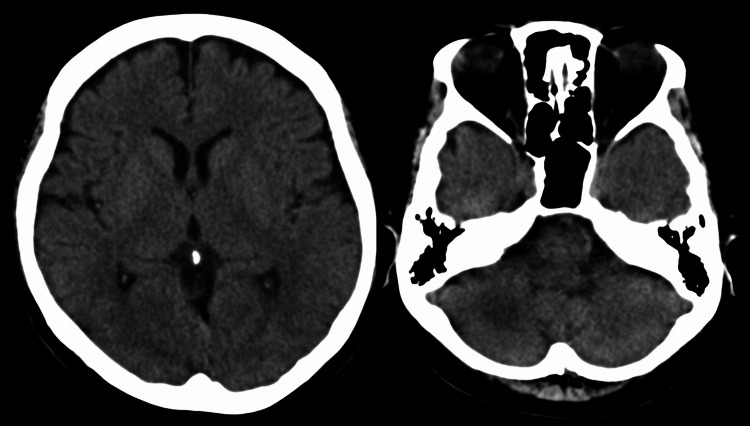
Computed tomography (CT) of the brain on admission.
